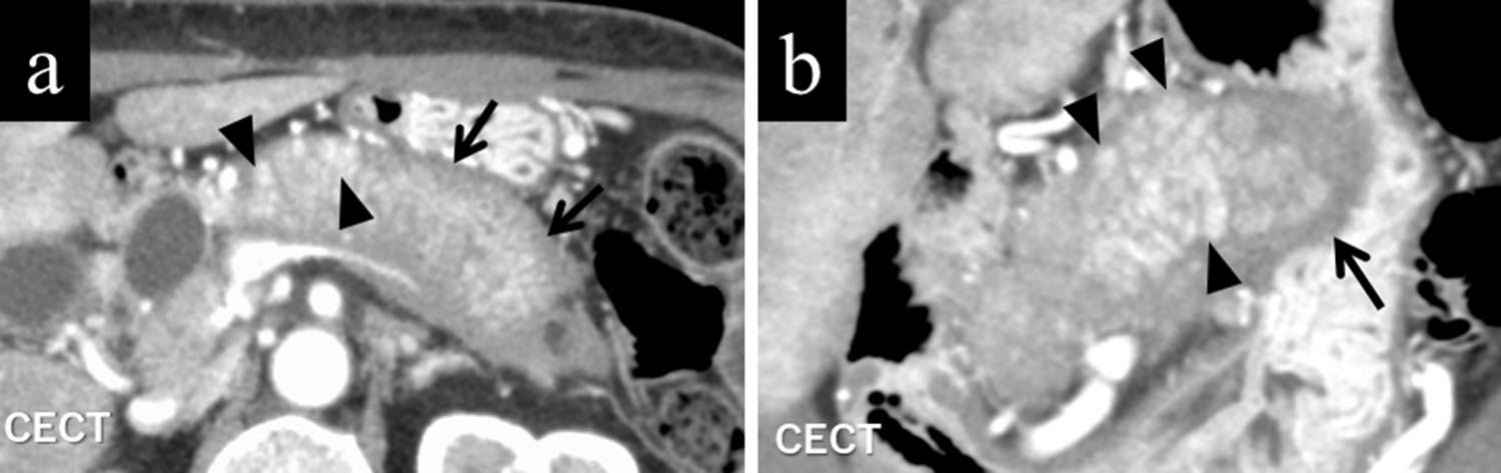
A female in her 60 s with typical autoimmune pancreatitis. a Axial contrast-enhanced CT in pancreatic phase. The pancreas is swollen as a whole, and punctate contrast-enhanced areas inside (arrowheads), as well as a typical band-shaped structure demarcating the lesion (arrows), are shown. b Coronal reconstruction of the same phase. Punctate contrast-enhanced areas (arrowheads) and a band-shaped structure surrounding the lesion (arrows) are also shown on the coronal image
